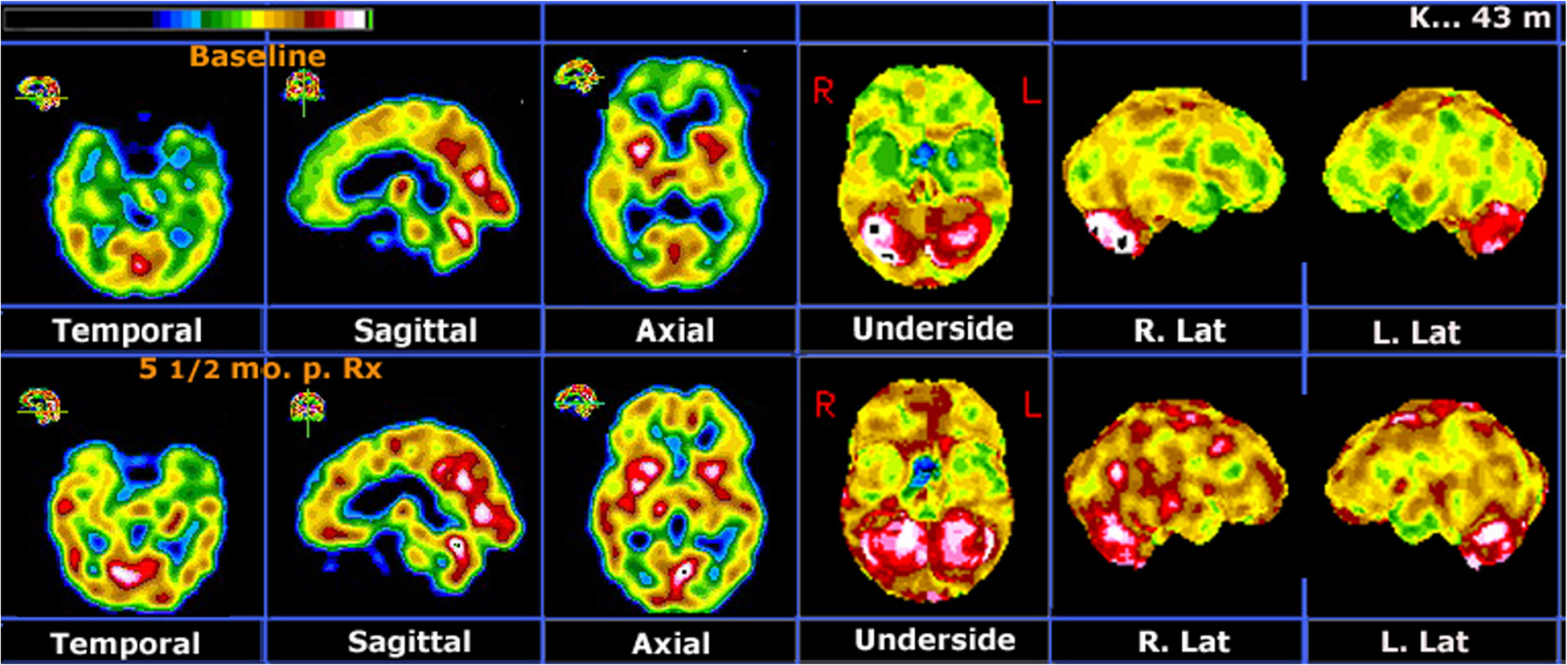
Representative images from the various types of displays used in our protocol. The first three images are part of the orthogonal displays and the last three are part of the stereotactic surface projections obtained with the Neurostat software (manufactured by Neurostat/3D-SSP, University of Washington, Seattle, WA, USA) [9]. Upper row: Baseline brain single-photon emission computer tomography (SPECT) shows multiple areas of hemispheric underperfusion, more accentuated in his frontal lobes, orbitofrontal areas and apico-mesial temporal areas. Marked hyperperfusion in his right putamen and in parts of his posterior cingulate and right cerebellum is also visible. Lower row: Follow-up SPECT scan at five and a half months post-treatment shows that practically all previously underperfused areas have significantly improved relative perfusion. Previously hyperperfused areas are either unchanged or increasingly hyperperfused.
